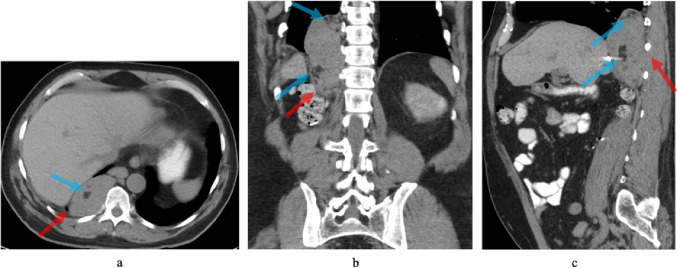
Subsequent axial (a), coronal (b) and sagittal (c) non-contrast CT images show a larger right retrocrural mass (red arrows) with fat containing components (blue arrows). The patient underwent surgical excision of the retroperitoneal enhancing masses and biopsy of the right retrocrural mass with a pathological diagnosis of angiomyolipoma
